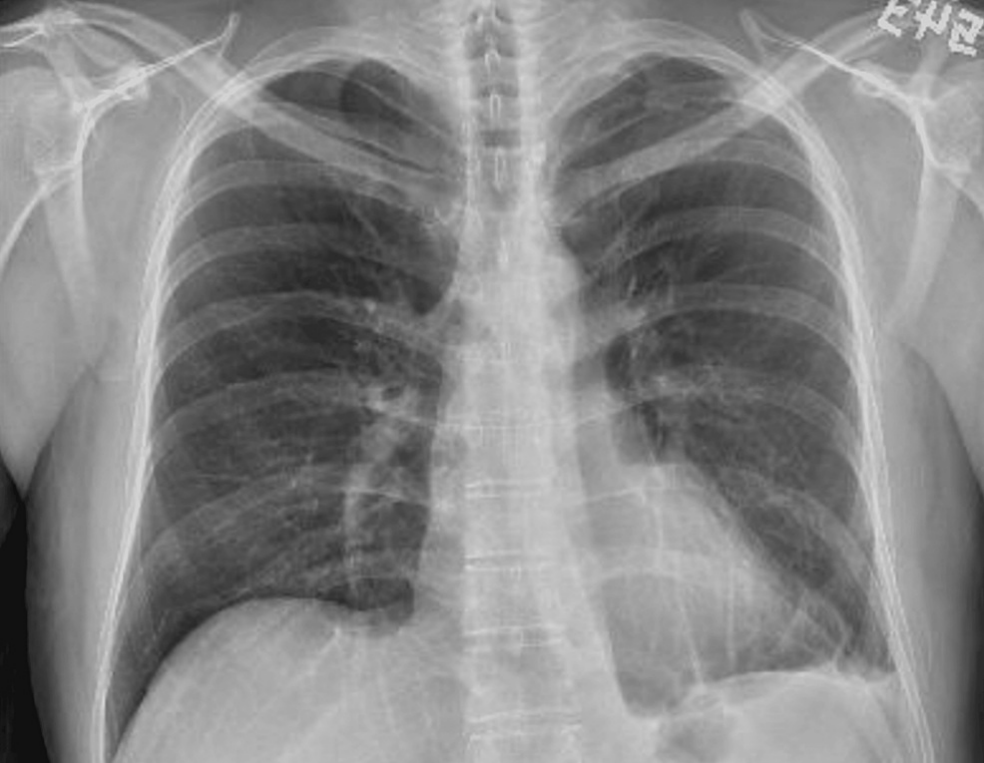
Follow-up chest X-ray with resolution of left-sided hemothorax.
